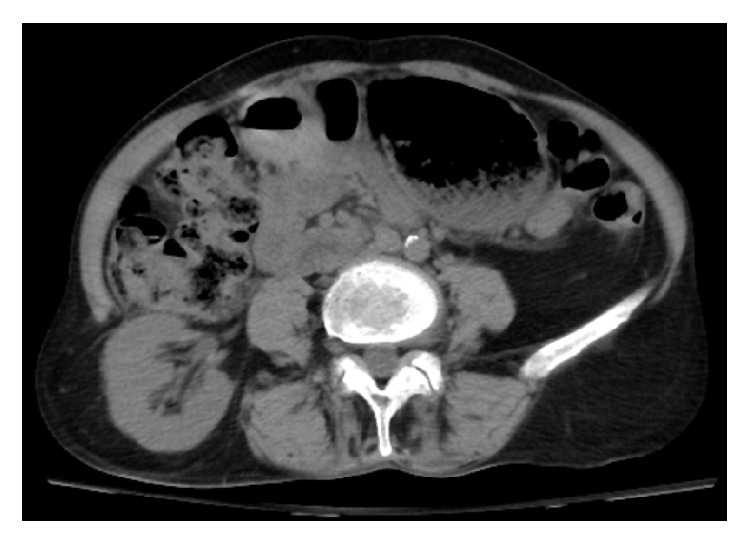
Axial CT demonstrates the extent of kidney herniation.
